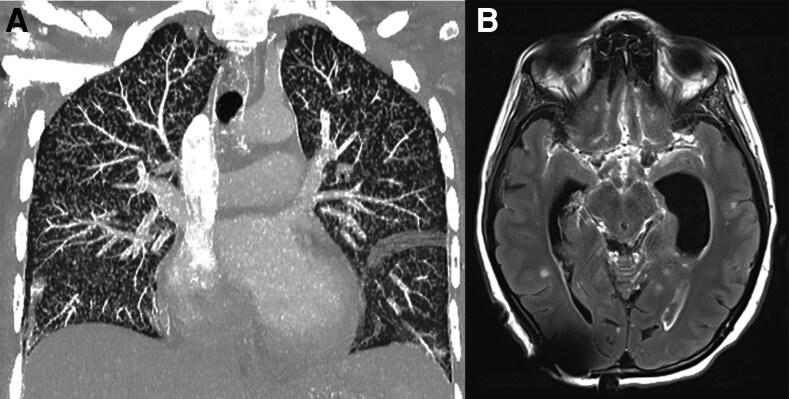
Computed tomography (CT) of the chest and magnetic resonance imaging (MRI) of the brain. *A*, Coronal chest CT scan demonstrating diffuse miliary pulmonary infiltrate. *B*, Axial fluid-attenuated inversion recovery MRI of the brain with contrast demonstrating multiple enhancing nodules in the leptomeninges of the basal cisterns and subcortical white matter, hydrocephalus of the temporal horn of the left lateral ventricle, and artefact in the parietal lobe from a ventriculoperitoneal (VP) shunt in the right lateral ventricle. The VP shunt into the left lateral ventricle was inserted later.
